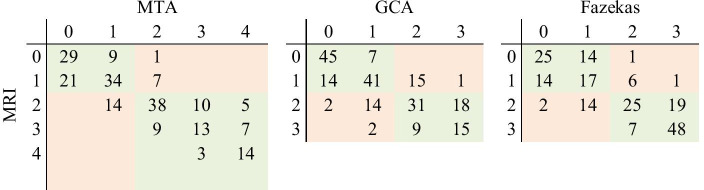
Confusion matrices for the computational MTA, GCA and Fazekas grades from MRI and CT. Green color indicates the number of correctly classified normal/abnormal subjects, and red color shows the number of incorrectly classified subjects
